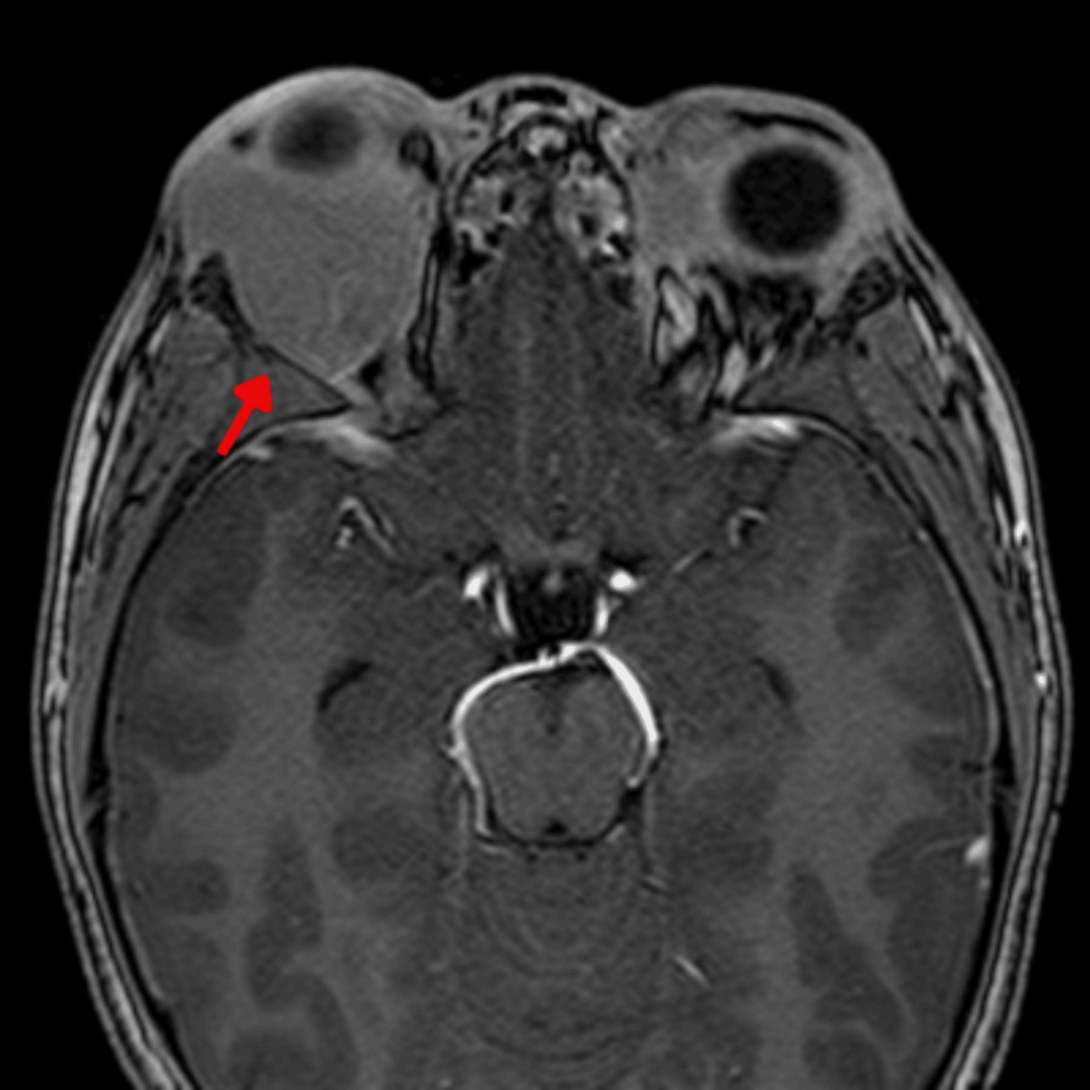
MRI in T1 phase with contrast showing an expansive right retroocular lesion (red arrow).
